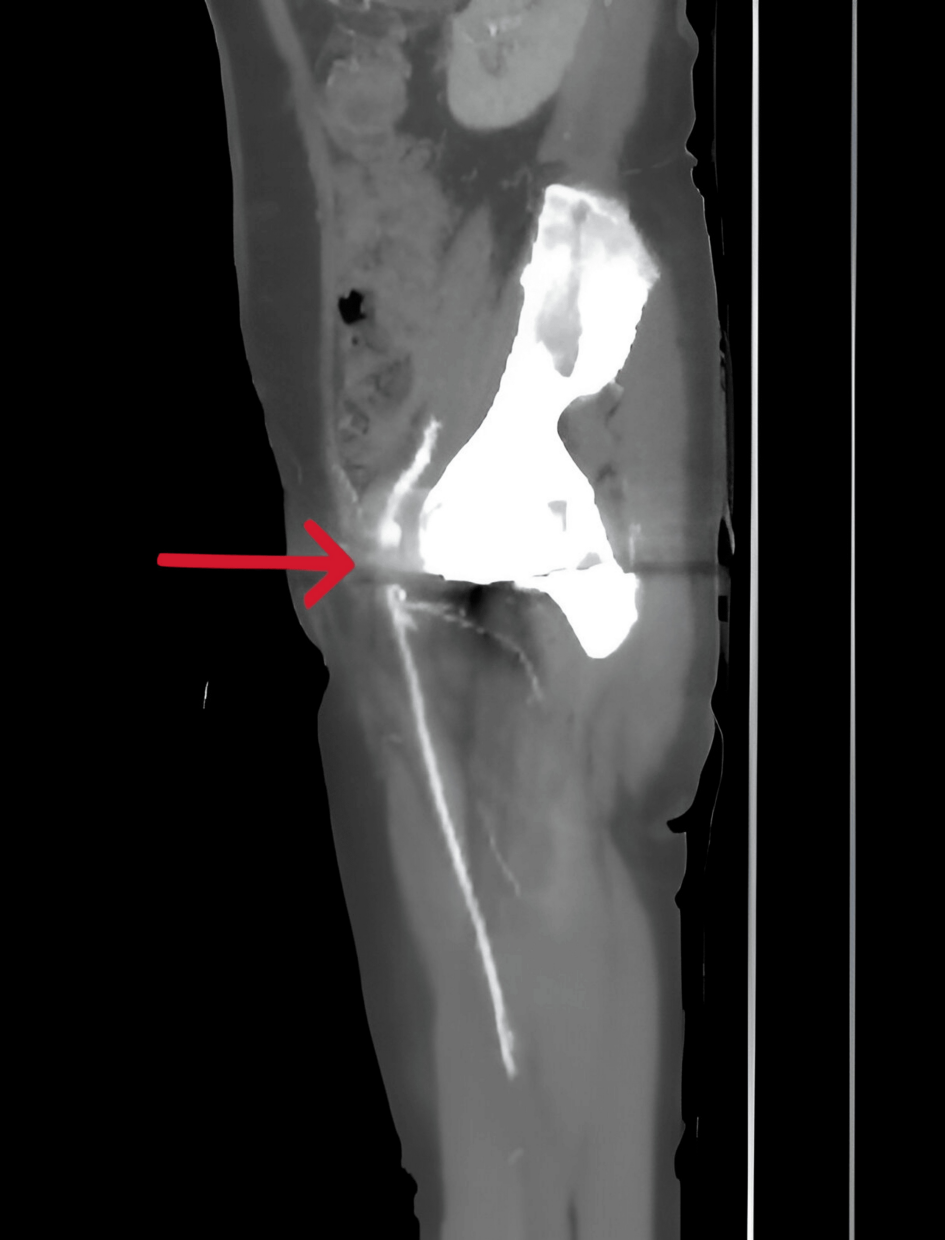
CT angiography of a right lower limb, which shows a non-opacification of a short segment (approximately 2 cm) of the right common femoral artery near its bifurcation (red arrow)
